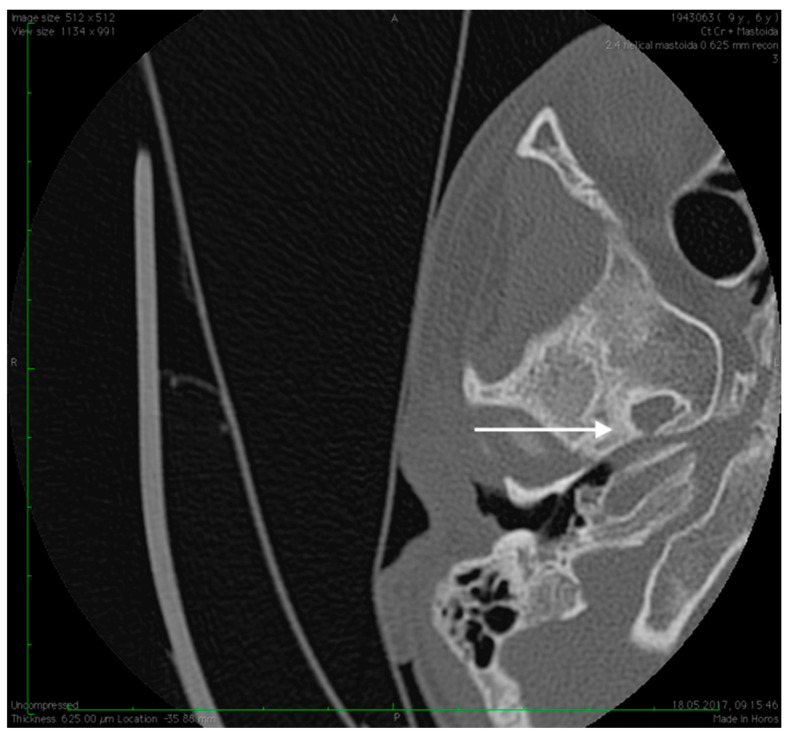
High resolution axial CT scan of right temporal bone exposing the missing foramen spinosum (arrow).
